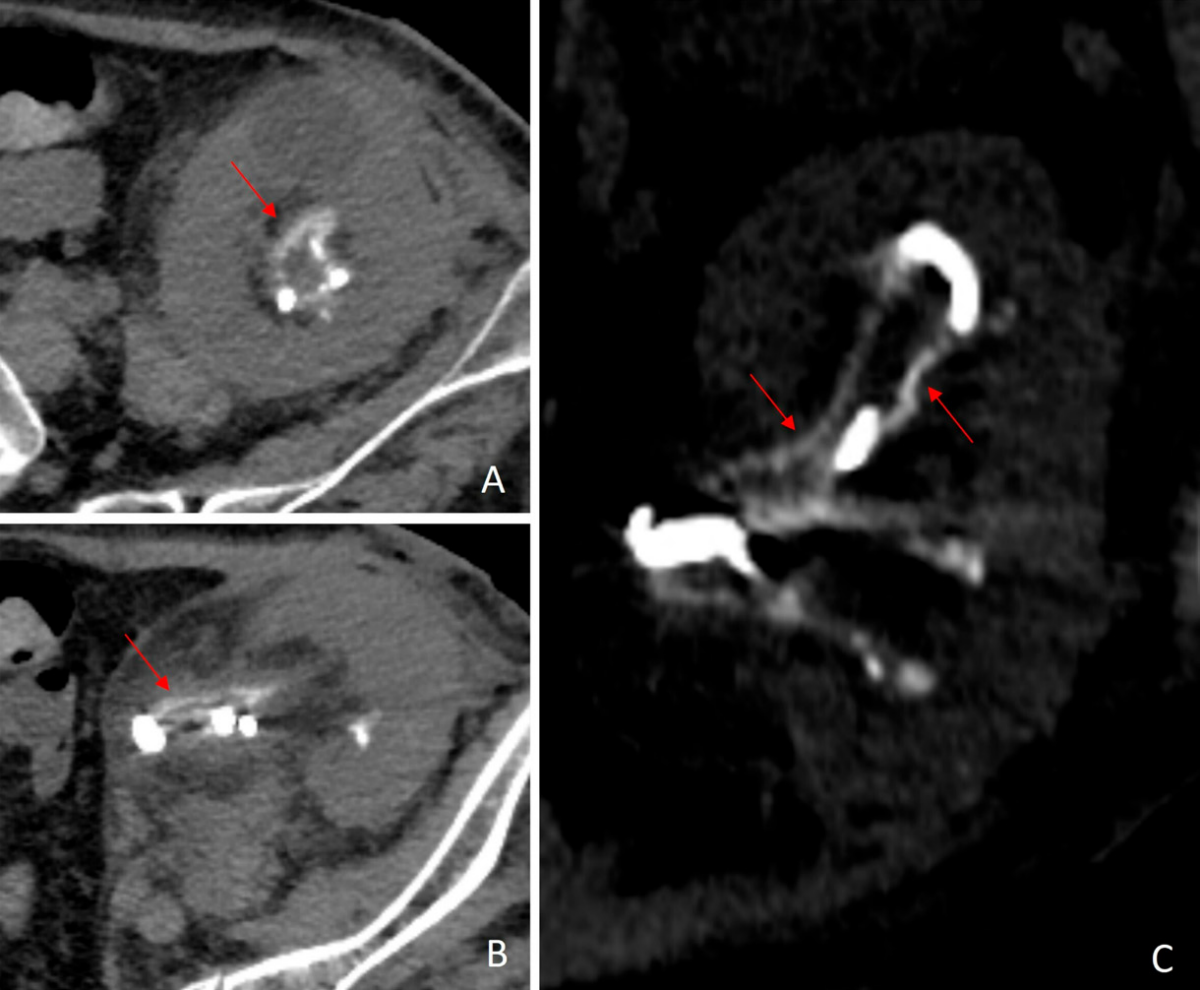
CT shows thick linear urothelial calcification (arrow), suggestice of alkaline-encrusted pyelitis. This condition is associated with an immunosuppressed state, previous urologic procedures, and pre-existing urothelial lesions.
